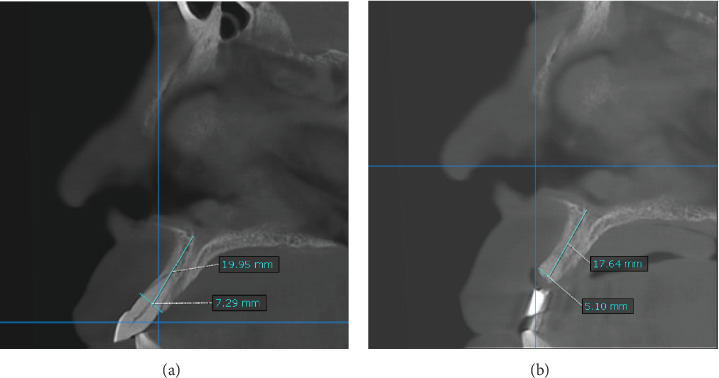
(a) Baseline measure CBCT of the lateral incisor (Bio-Col technique). (b) Measure of 6 months of healing.
